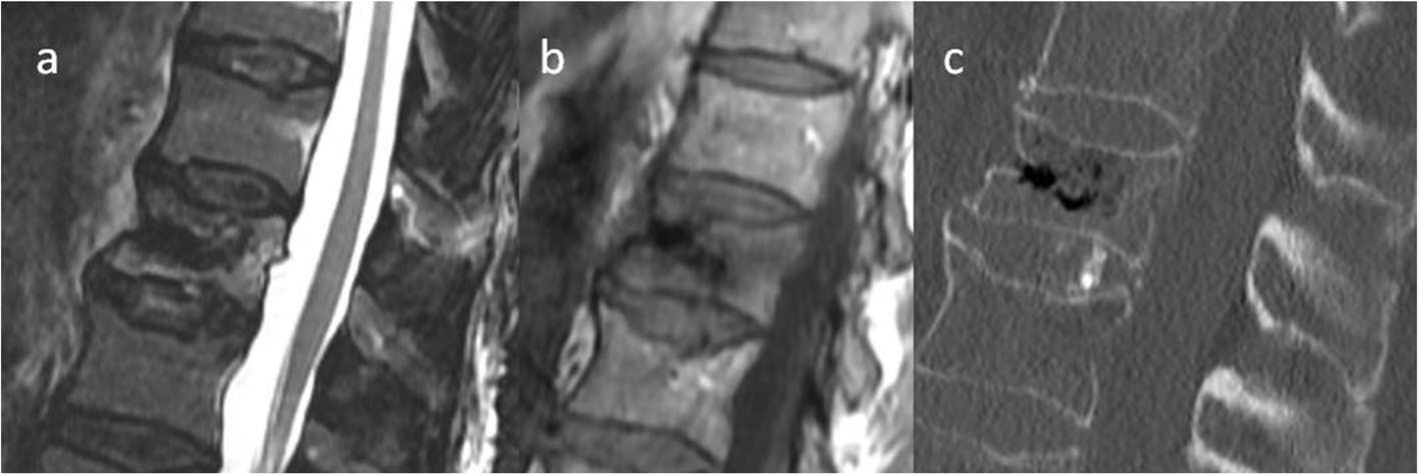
Depicted is a case with high disagreement between the classification done by MRI (a: STIR-Sequence, b: T2-Sequence) only and under consideration of both MRI and CT (c). The majority of rater classified this fracture as type OF 4 fracture after evaluating the MRI and re-classified it as type OF 3 fracture under consideration of the additional CT. However, the OF score was 7 and 9 in case of OF type 3 and OF type 4 fracture, respectively. Thus, operative treatment was recommended based on the OF-score regardless of type OF 3 or 4 fracture classification
